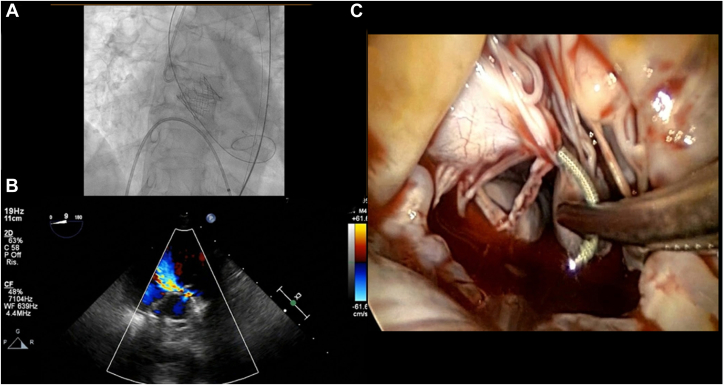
Fluoroscopic, Echocardiographic, and Intraoperative Findings of Mitral Valve Guidewire Entrapment (A) Fluoroscopic check of the positioning result of a 23-mm Sapien 3 Ultra valve (Edwards Lifesciences); evidence of anomalous movement of the Safari guidewire. (B) Transesophageal echocardiography imaging of highly eccentric mitral regurgitation jet caused by anterior mitral regurgitation jet caused by anterior mitral leaflet retraction. (C) Intraoperative endoscopic visualization of Safari guidewire entrapment within the mitral subvalvular apparatus.
